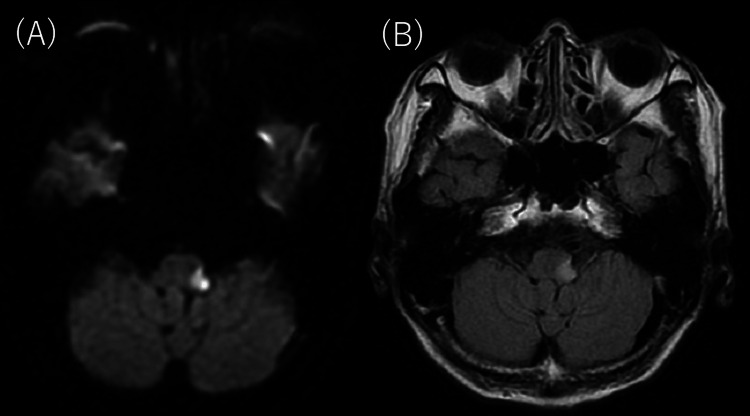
The results of brain magnetic resonance imaging (MRI). (A) Diffusion-weighted brain MRI showing hyperintensity in the left lateral medulla oblongata. (B) T2-weighted fluid-attenuated inversion recovery (T2-FLAIR) brain MRI showing hyperintensity in areas that were hyperintense on diffusion-weighted imaging.
